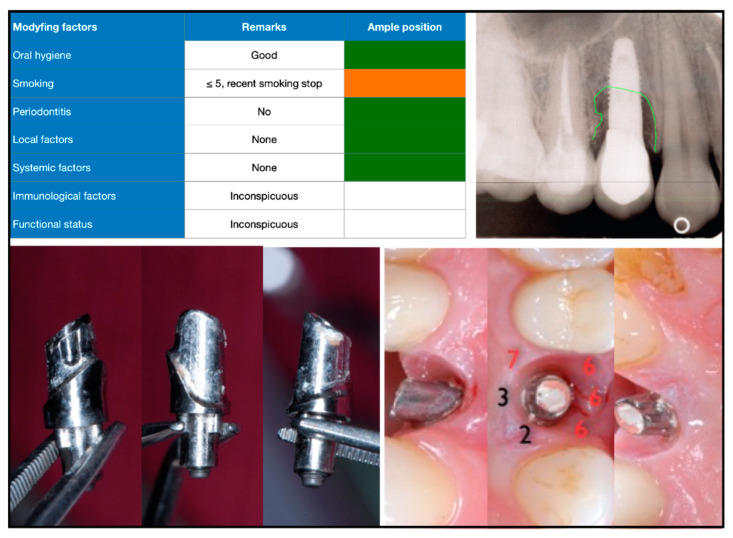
Initial findings in patient with healthy medical history (risk profile, top left). Tooth 14 was removed due to endodontic complication and ridge preservation was performed at that time. Three months later, implant placement was performed. Implant crown was cemented intraorally on an individualized abutment. Four years after placement, clear peri-implant bone resorption can be seen in radiograph (top right). Bottom left: removed abutment; bottom right: clinical findings of peri-implant mucosa with cement residue on abutment.
